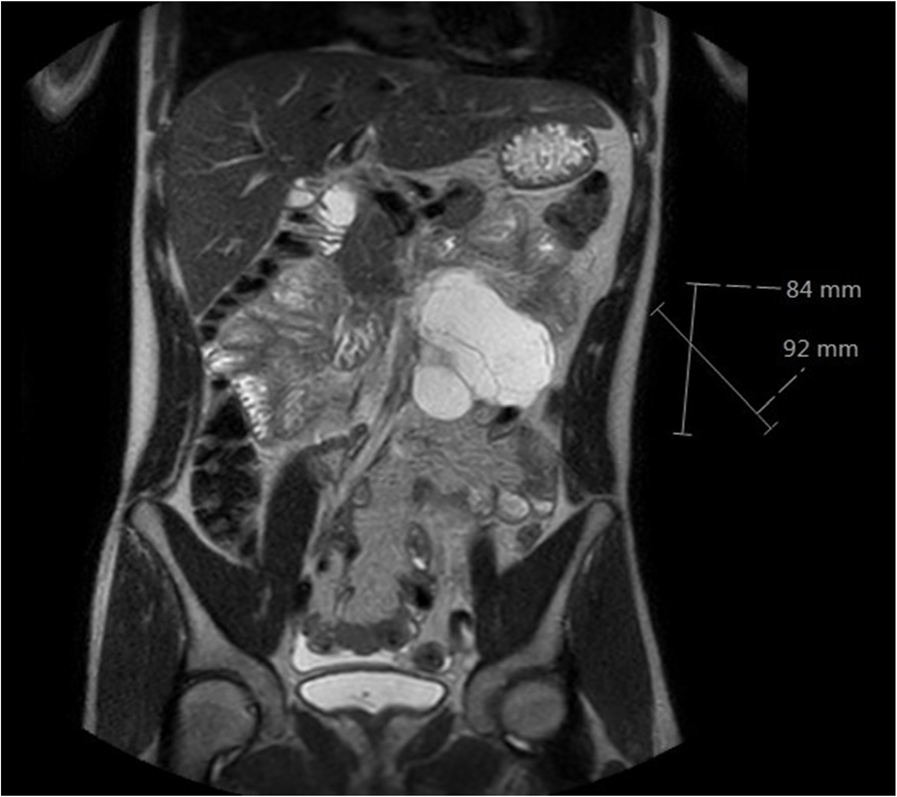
Left-sided multi-chambered intraabdominal lymphocele arising after post-chemotherapy RPLND. Magnetic resonance imaging, T2-weighted imaging, coronal sectioning. This lymphocele resolved spontaneously within 3 months
